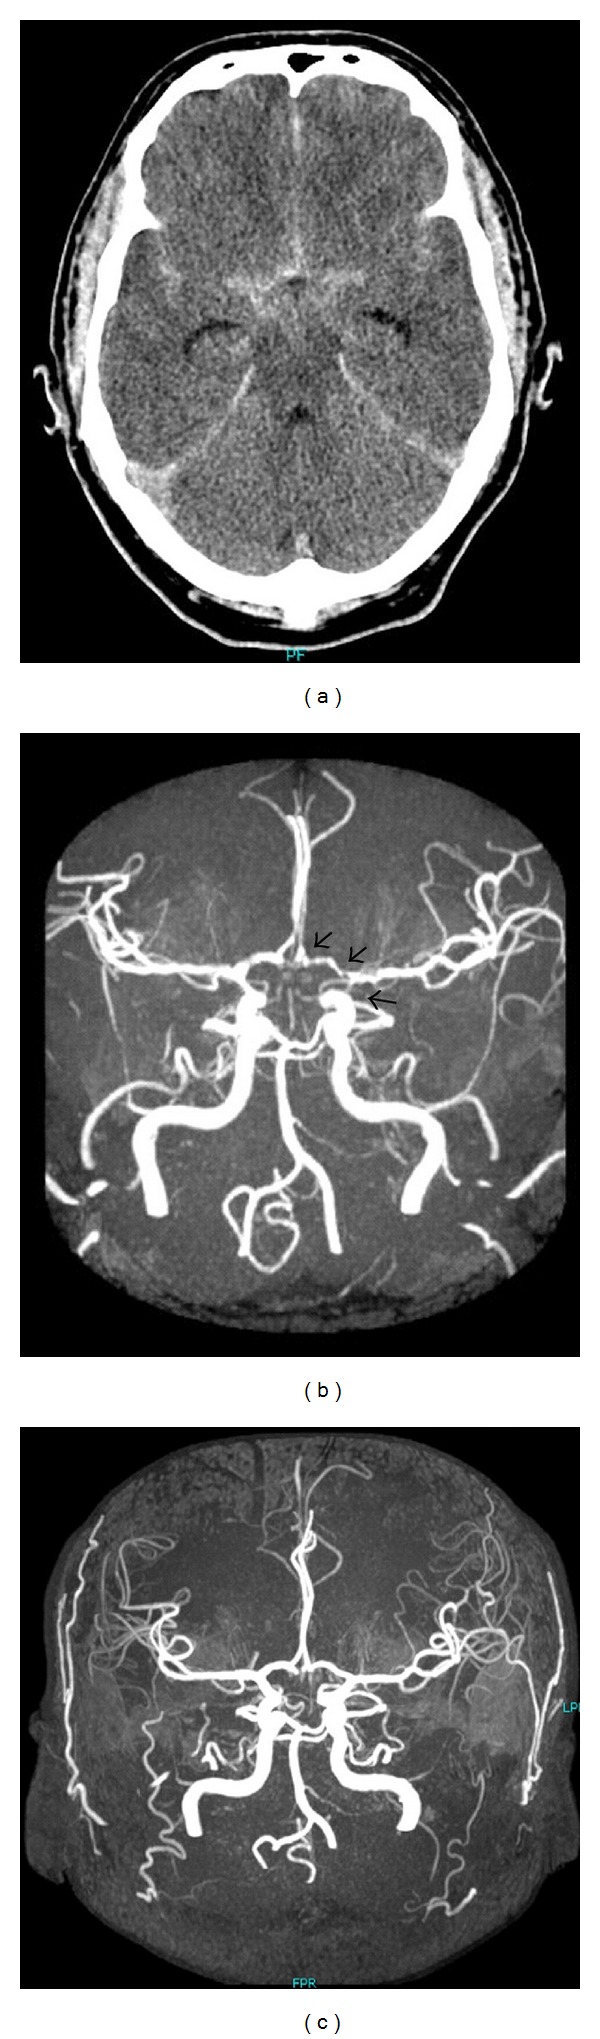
(a) initial CT scan shows SAH; (b) MRA shows radiologic CVS of left-sided ACA and ICA (arrows); (c) 6-month followup MRA shows normalized vascular patterns.
